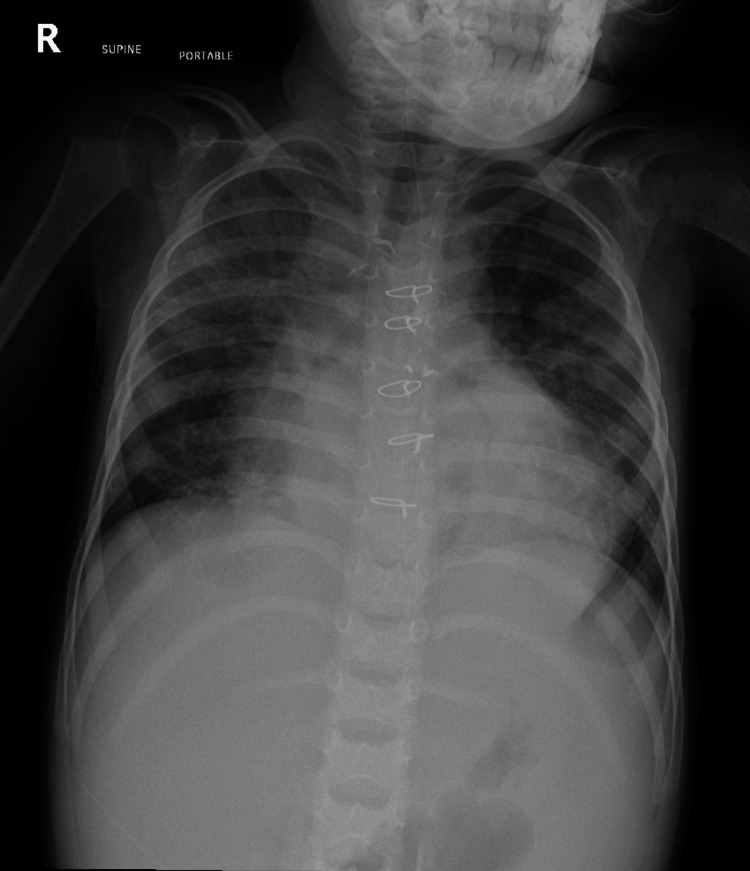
Chest X-ray taken at first presentation showing cardiomegaly, pneumonic changes, and increased pulmonary vascular margins.
